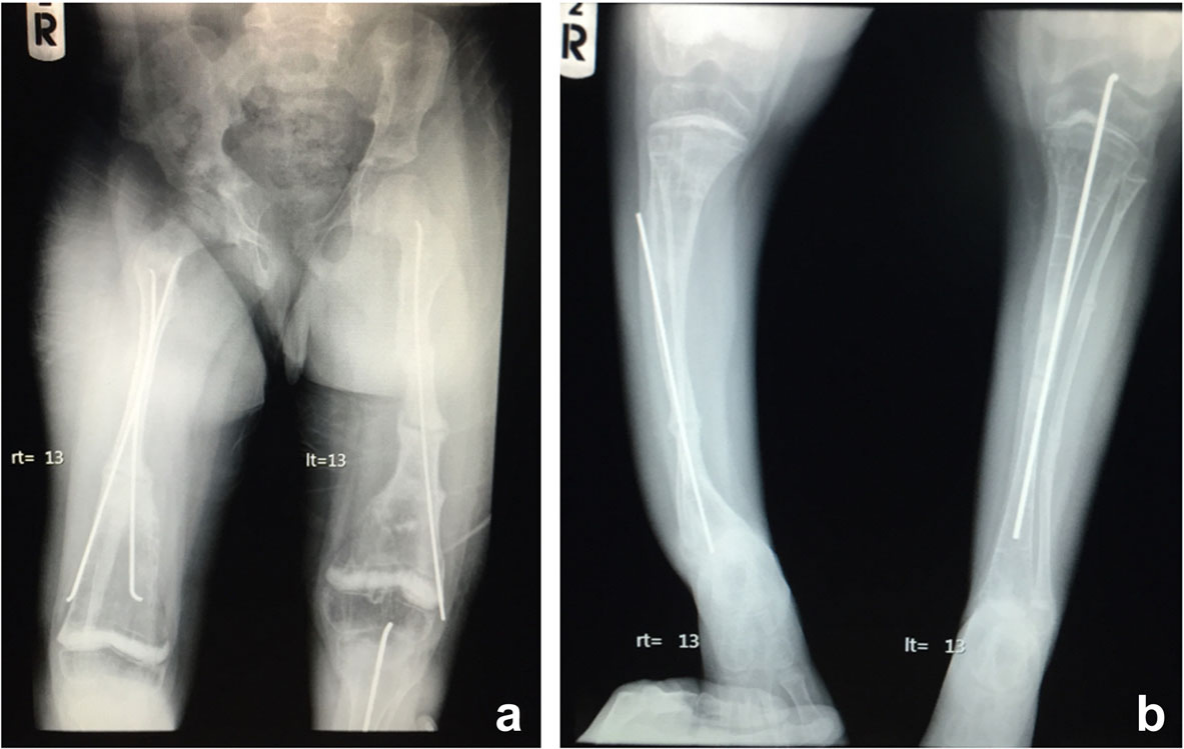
AP plain radiographs of lower extremities at the age of nine. a Both femoral AP radiographs reveal a nonunion at the shaft with shortening and varus deformity of the left femur. Intramedullary rods were used to stabilize the fractures. Generalized osteopenia is noted. b Both tibial AP radiographs reveal a nonunion, varus deformity, and shortening of the right tibia. Intramedullary rods were used to stabilize the fractures
